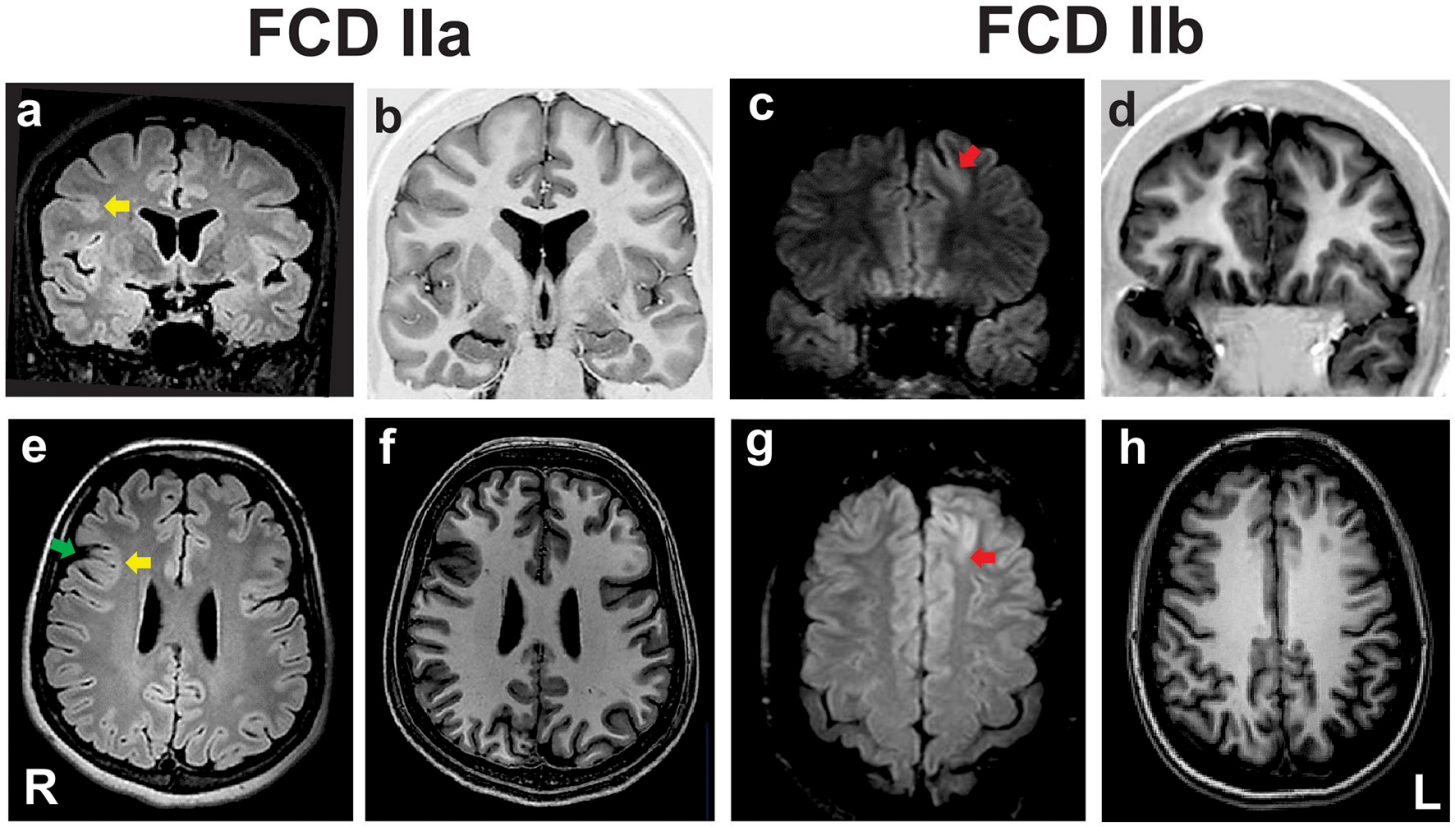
Preoperative coronal fluid-attenuated inversion recovery [FLAIR; (a, c)] and T1 inversion recovery (b, d), and axial FLAIR (e, g) and T1-weighted images (f, h) of two patients with pharmacoresistant frontal lobe epilepsy whose surgical samples showed focal cortical dysplasia (FCD) ILAE type IIa (a, b, e, f) and FCD type IIb (c, d, g, h). In the patient with FCD IIa there is a discrete hyperintense signal of gray matter in T2/FLAIR [yellow arrows in (a, e)], mild blurring of the gray-white matter junction in T1 scans, and a cortical dimple indicated by the green arrow in (e). The patient with FCD type IIb has a deep sulcus with a mildly thickened cortex and hyperintense FLAIR signal in the left frontal lobe [red arrows in (c, g)]. ILAE, International League Against Epilepsy; L, left side; R, right side.
